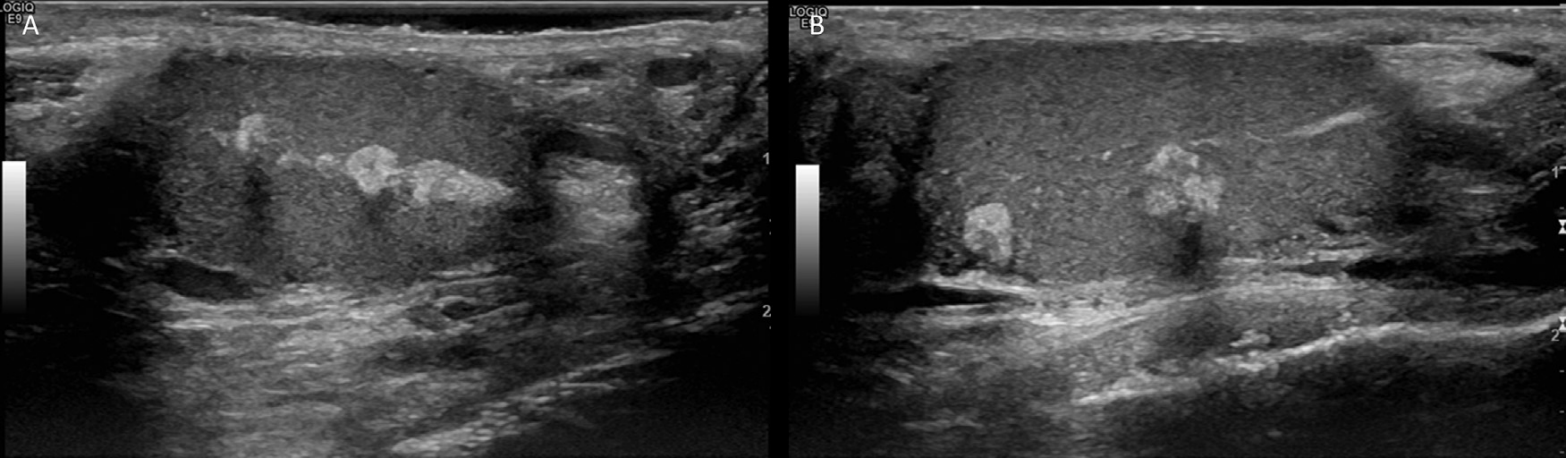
Follow up transverse testicular ultrasound in 3 mo, Right (A) and Left (B), displays unchanged size of the small mixed echogenicity masses in the bilateral testes.
